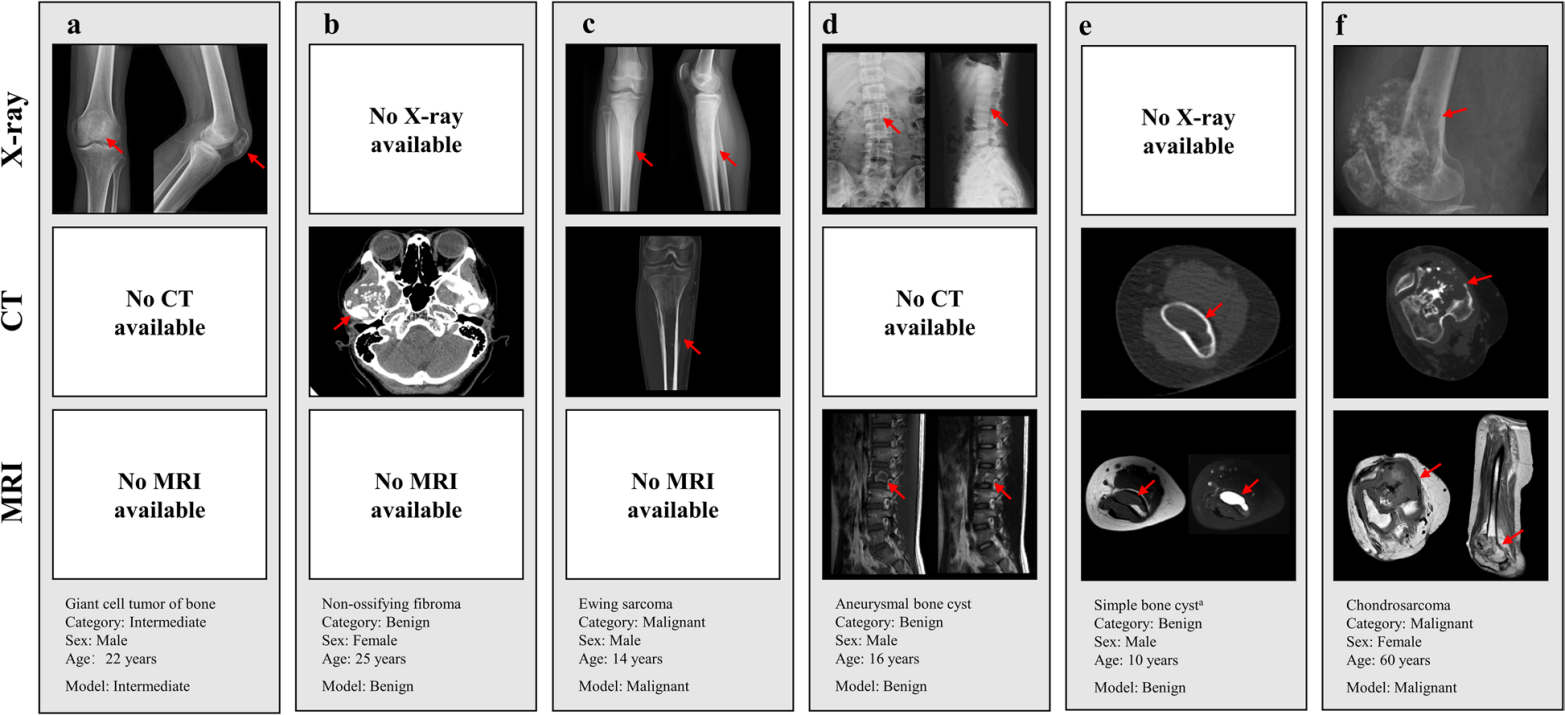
Representative examples (red arrow) correctly classified by the PBTC-TransNet fusion model on the external test set. ^a^T2-weighted image (T2WI) with fat-suppression is provided to the model when T2WI was not available. PBTC-TransNet, Primary Bone Tumor Classification Transformer Network
